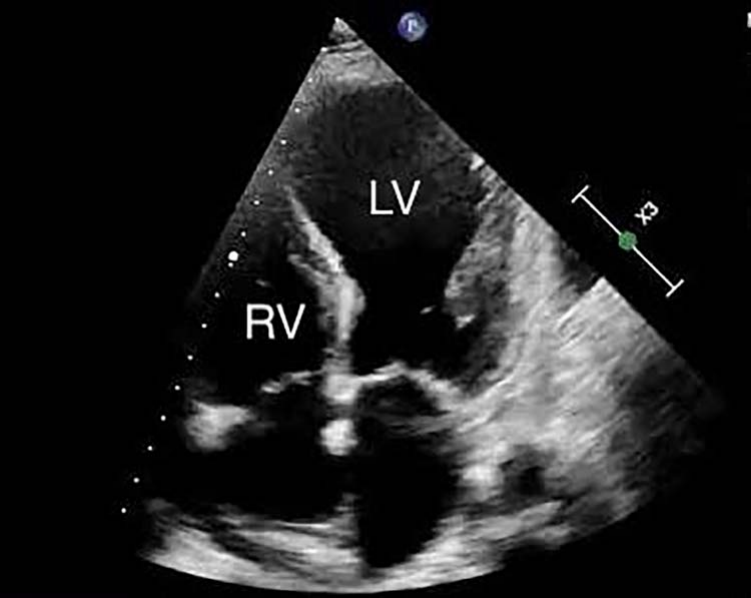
Apical 4 chamber view of left ventricular and right ventricular views shows severe segmental systolic dysfunction. Estimated LVEF was 20% by Simpson’s biplane method.
